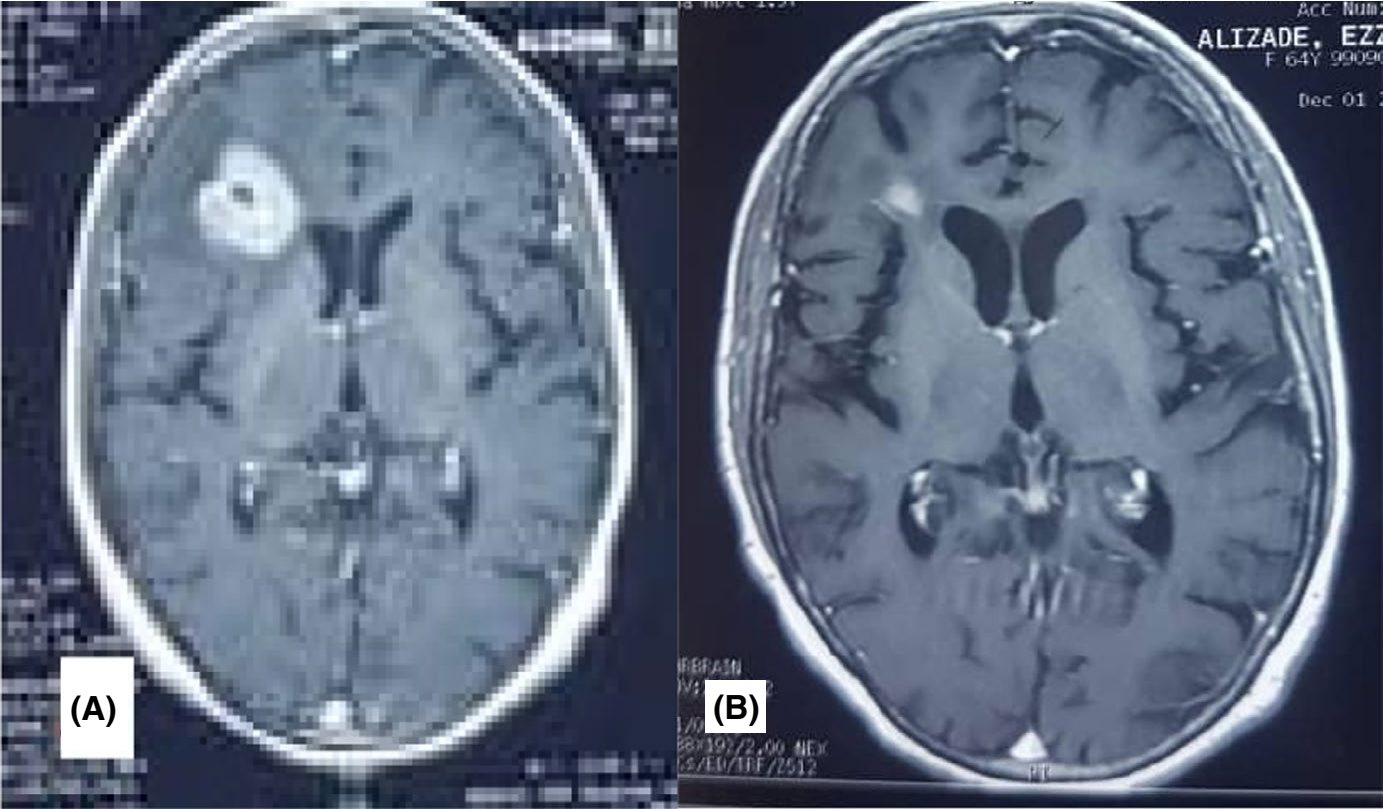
Post‐contrast axial brain MRI. (A) brain MRI before treatment. An enhancing lesion measuring 3 cm is present in the right frontal lobe. (B) 3 months after treatment. A subcentimeter enhancing lesion is visible in right frontal lobe
